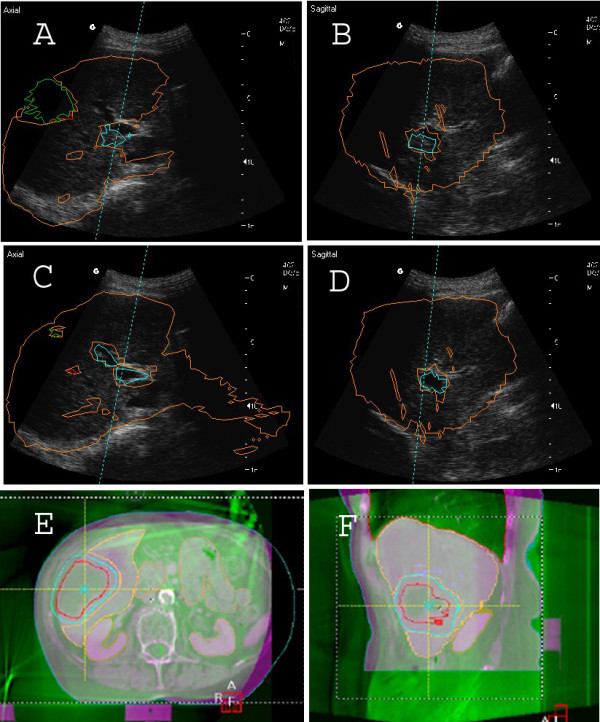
Daily positioning with BAT®. Before (A-B) and after (C-D) position correction. Surrogate structures and PTV are marked with colors: orange, liver; green, PTV; blue, portal vein; red, liver veins. E-F: Alternative repeat breath-hold positioning with CBCT. Green, CBCT; magenta, planning-CT.
